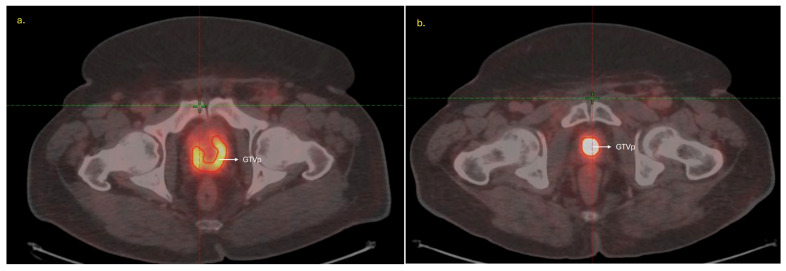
Representative PSMA-PET/CT images showing the gross tumor volume of the prostate (GTVp) delineated on (a) pre-treatment and (b) post-treatment registered scans in a patient included in the delta-radiomics analysis.
